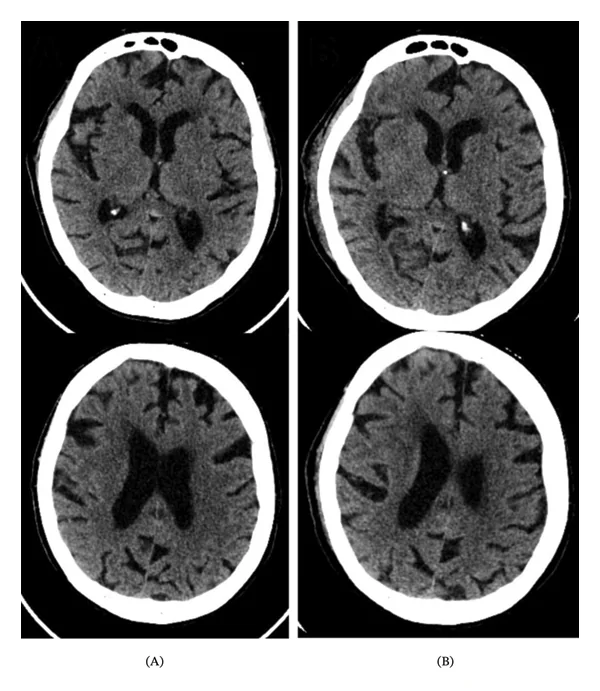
Noncontrast CT on admission (A) and 24 h after reperfusion therapy (B). No hemorrhagic transformation or visible infarction is seen on follow‐up CT.
